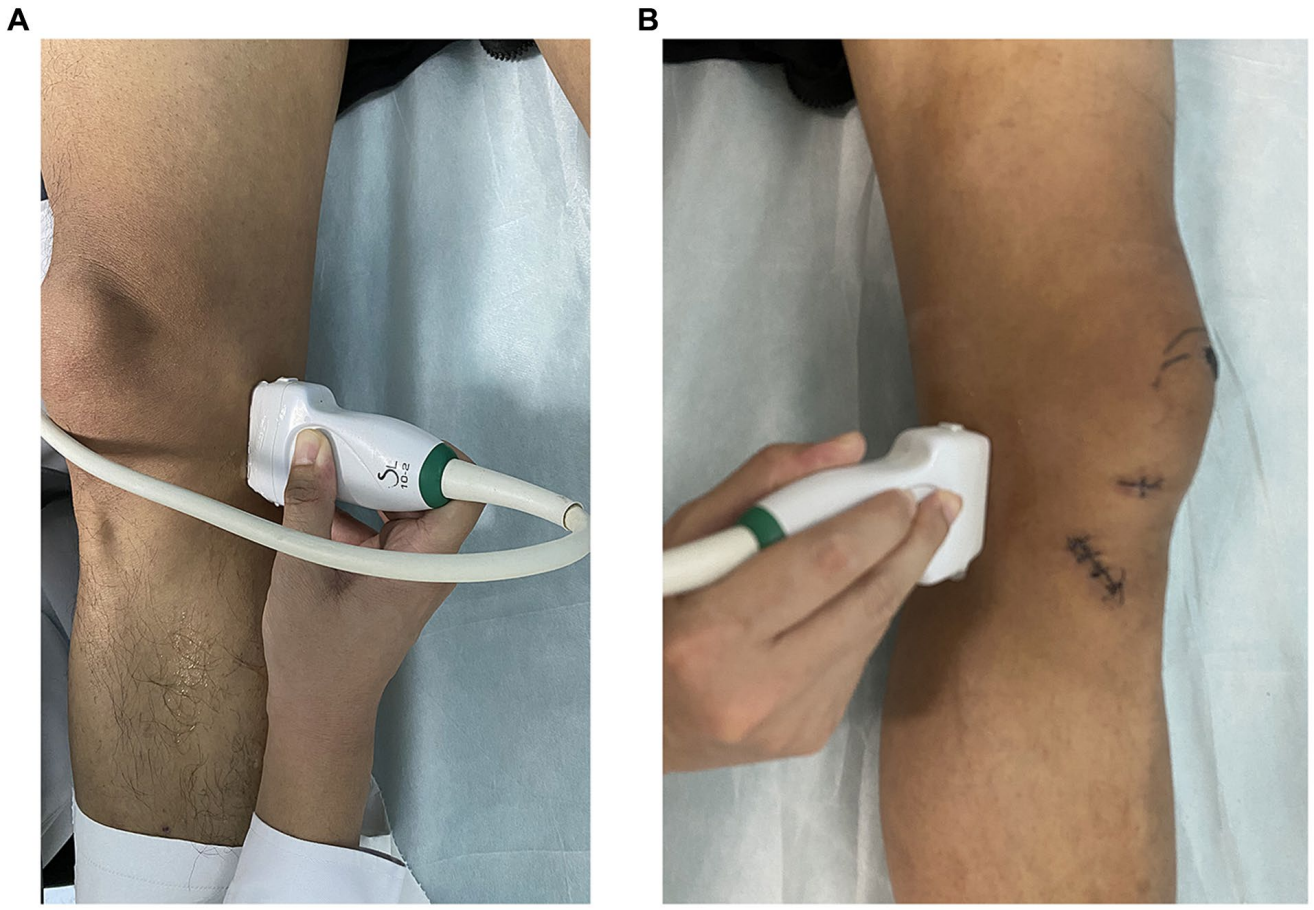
Ultrasonic shear wave elastography operation. (A) Ultrasound measurement of meniscus in a supine position with knee joint in an unloaded state. (B) Measurement of meniscus position in supine position using ultrasound in patients after knee joint surgery.
